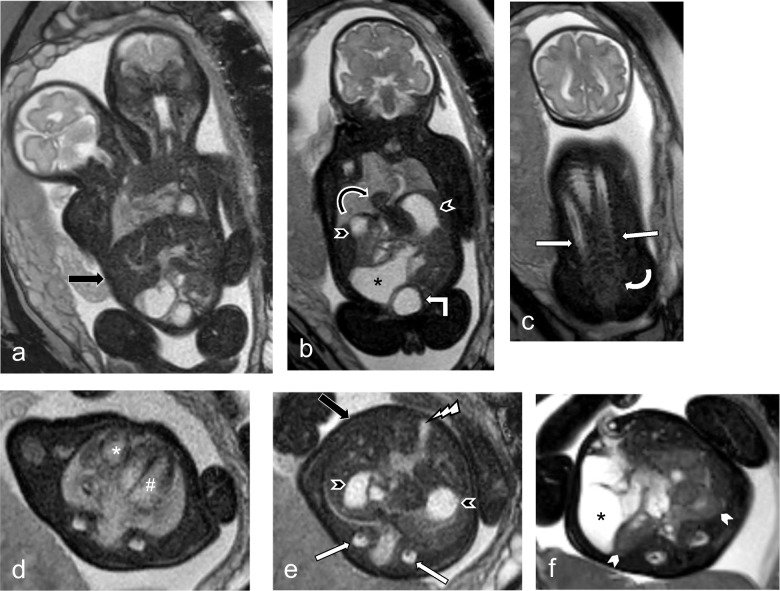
Fetal MRI of dicephalic parapagus at gestational age 32 weeks, 1 day, showing lateral fusion of the torso with separate heads. a-c BTFE sequential ventral-to-dorsal coronal images show the shared liver (*black arrow*), separate stomachs (*black arrowheads*), single urinary bladder (*white right-angle arrow*), and two spinal canals (*white arrows*) with osseous fusion at the level of the pelvis (*curved white arrow*). There is also cephalad protrusion of a small midline component of the shared liver representing a small diaphragmatic hernia or eventration (*curved black arrow*) and a dilated fluid-filled loop of bowel in the right lower quadrant (*black asterisk*), raising concern for bowel atresia or midgut volvulus. d BTFE axial image through the shared chest shows two asymmetric side-by-side hearts (*white asterisk* and *white pound sign*). The smaller right-sided heart (*white asterisk*) was shown to have a single ventricle on fetal echocardiography. e BTFE axial image through the upper abdomen shows the shared liver (*black arrow*), single gallbladder (*white lightning bolt*), separate stomachs (*black arrowheads*), and two spinal canals (*white arrows*). f BTFE axial image through the lower abdomen shows two kidneys (*white arrowheads*) and the dilated bowel loop (*black asterisk*) also depicted in b. *BTFE*, balanced turbo-field echo
